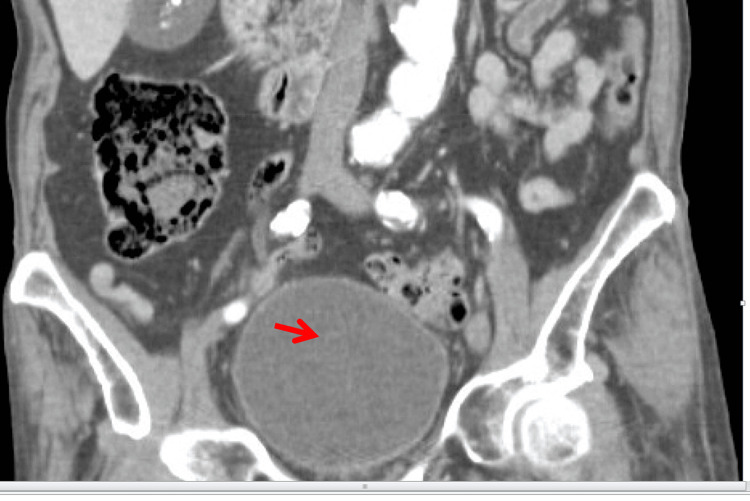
CT abdomen and pelvis, coronal view. Distended urinary bladder (arrow), consistent with bladder outlet obstruction
